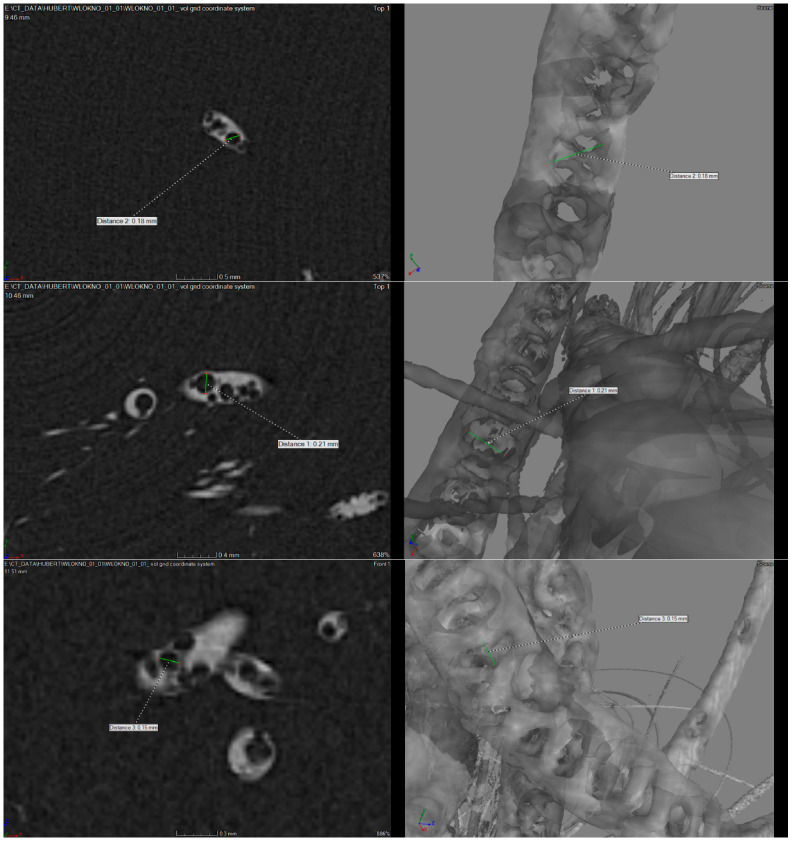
High-resolution X-ray scans revealing the internal structure of the fibers and confirming the location of the modifiers.
